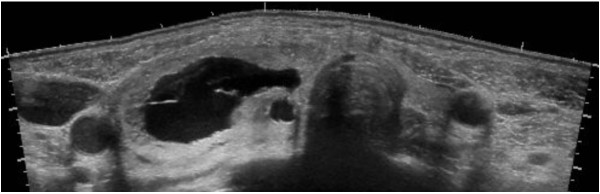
Ultrasonographic findings. Thyroid ultrasonogram demonstrates a 4.6 × 5.1 × 2.3 cm cystic dominant nodule in the lower portion of the right thyroid lobe and atrophy of the contralateral lobe.
